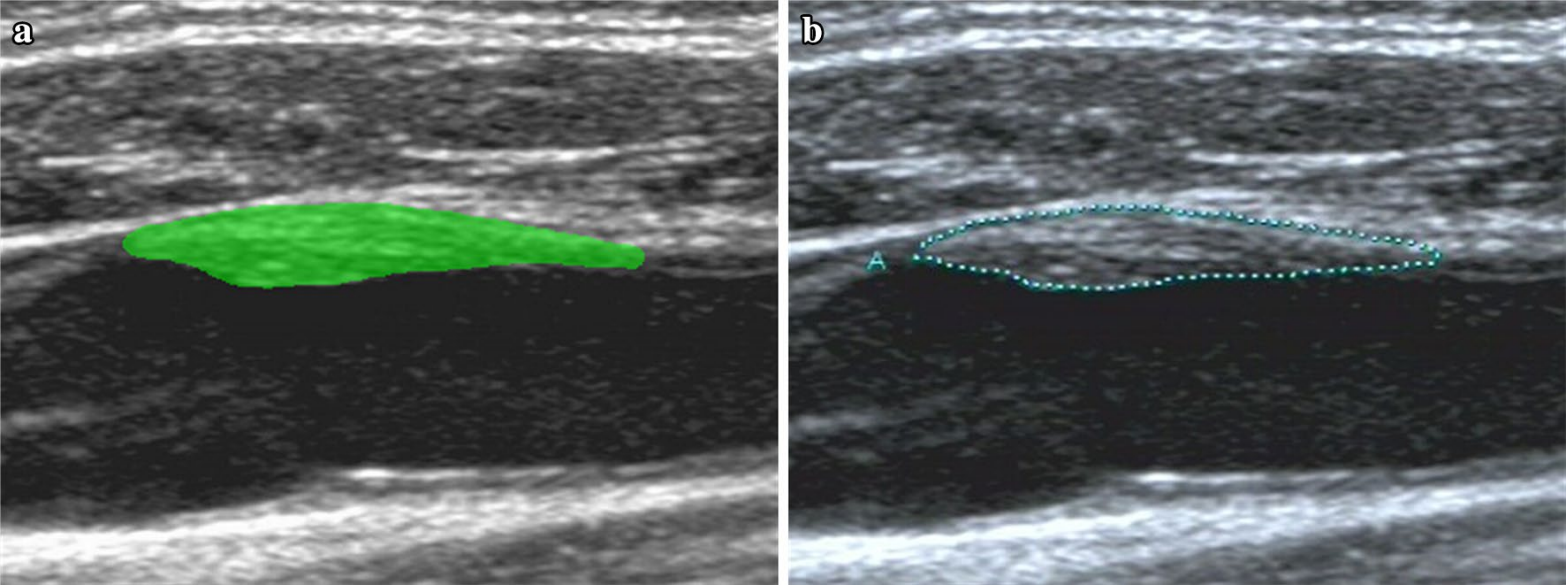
The region of interest selection. a The region of interest selection according to (b) using MaZda software. b Plaque defined by a specialized physician with 5 years vascular ultrasound working experience
